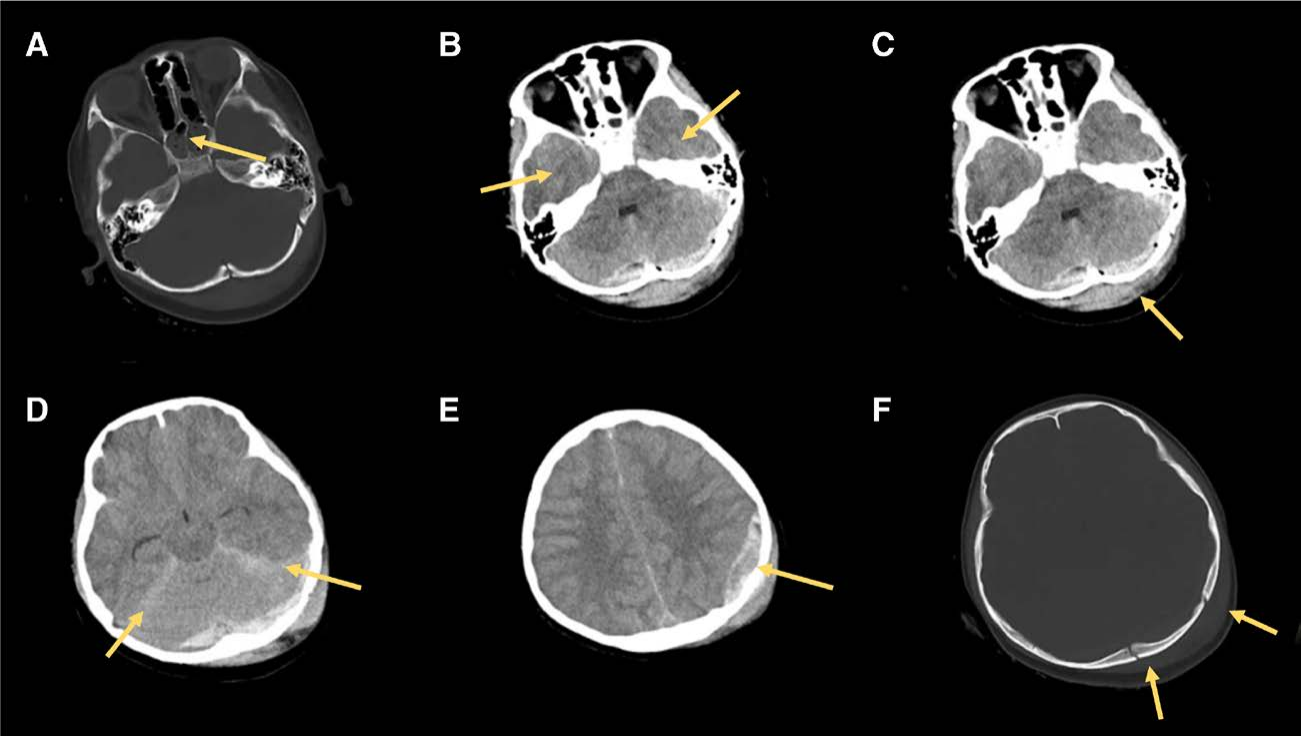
CT scan of the head. (A) Irregularities in the cortex of the right and left lateral walls of the pterygoid mass and the body wall of the pterygoid sinus, consider fracture, pterygoid sinus effusion; (B) mottled hyperdense shadows in the temporal lobes bilaterally; (C) bilateral occipital subdural hematomas, left paraventricular subcortical soft tissue injuries and hematomas; (D) subarachnoid hemorrhage: bilateral occipital subdural hematomas, left parietal occipital subcortical soft tissue injuries and hematoma formation; (E) left parietal epidural hematoma; (F) left occipital multiple fractures, left parietal bone fracture.
